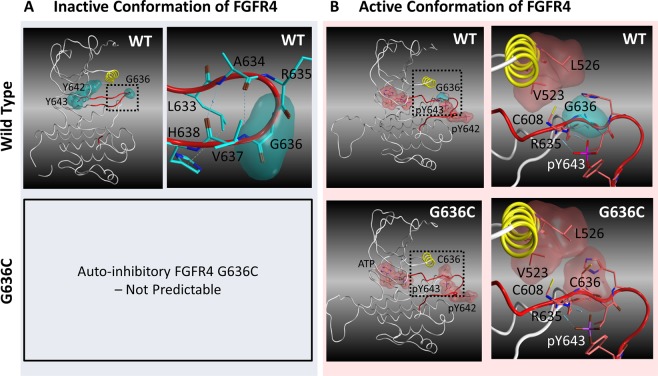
Three-dimensional structural models of catalytically inactive and active FGFR4. (A) X-ray structure of the kinase domain of FGFR4 in the auto-inhibitory conformation (PDB code: 4TYE^20^). The αC helix and A-loop are yellow and red, respectively. Blue and yellow dotted lines represent hydrogen bonds and the CH-π bond, respectively. The upper left panel shows an overview of the kinase domain, and the upper right panel shows the amino acid resides within 4.5 angstroms from G636. (B) Predicted 3D structure of the catalytically active conformation kinase domain. The left panels show overviews of the kinase domain, and the right panels show the amino acid resides within 4.5 angstroms from G636 or C636.
